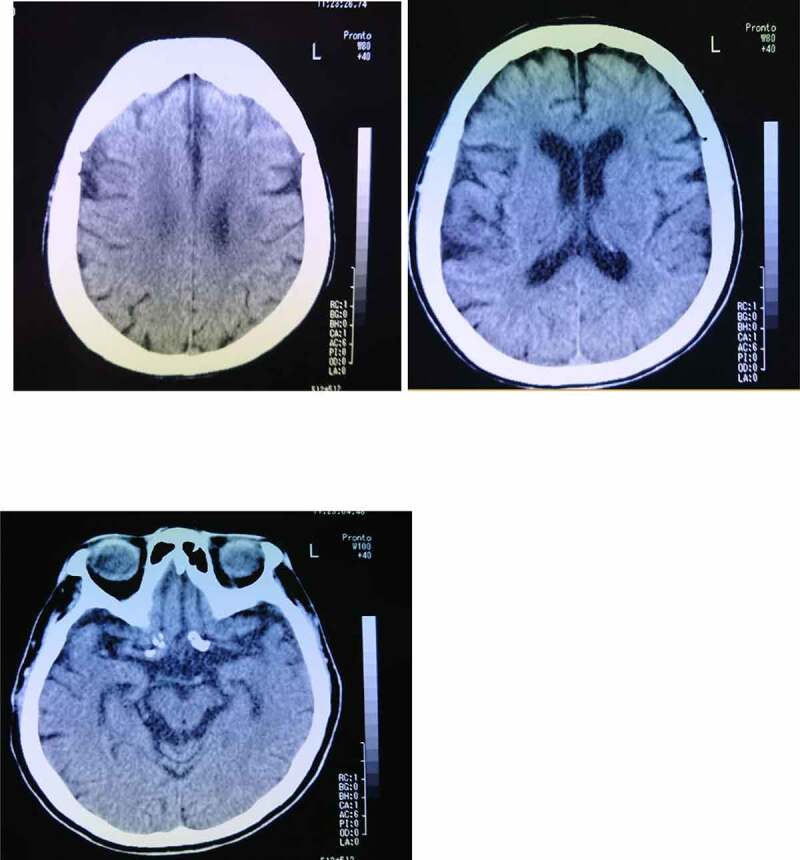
Brain computed tomography image of an 84-year-old woman shows mild cortical atrophy in the hippocampus and the absence of a cerebrovascular lesion.
